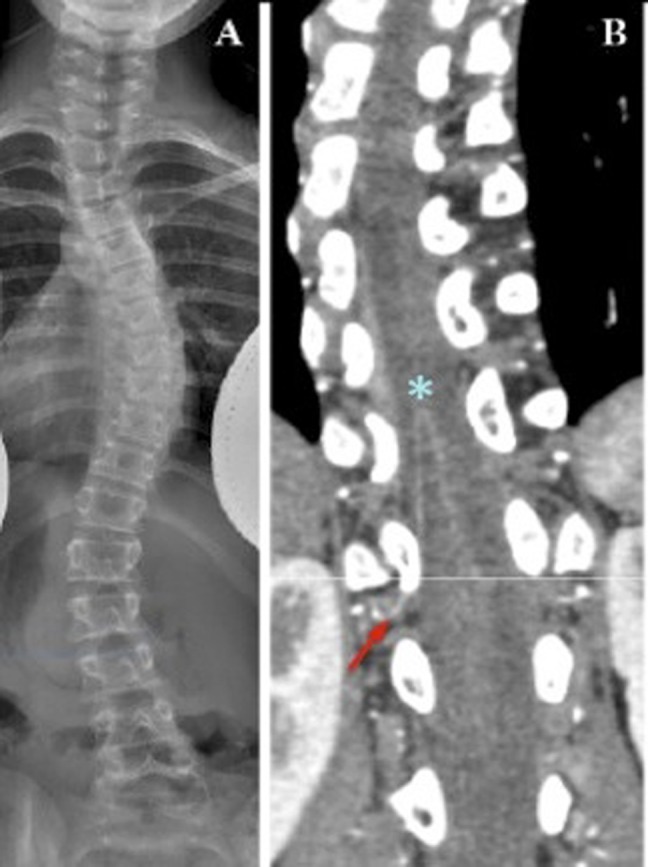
Radiograph showing a 10-year-old premenarcheal girl with progressive thoracic more than lumbar scoliosis despite bracing treatment (A) was scheduled to undergo selective convex T5-12 anterior spine instrumentation surgery. A preoperative CT angiography (B) demonstrated a dominant artery emerging from the right T12 segmental (red arrow) and continuing to the anterior spinal artery in a “hairpin loop” (blue asterisk). Instrumentation was modified to avoid the dominant artery at the distal-most planned level.
